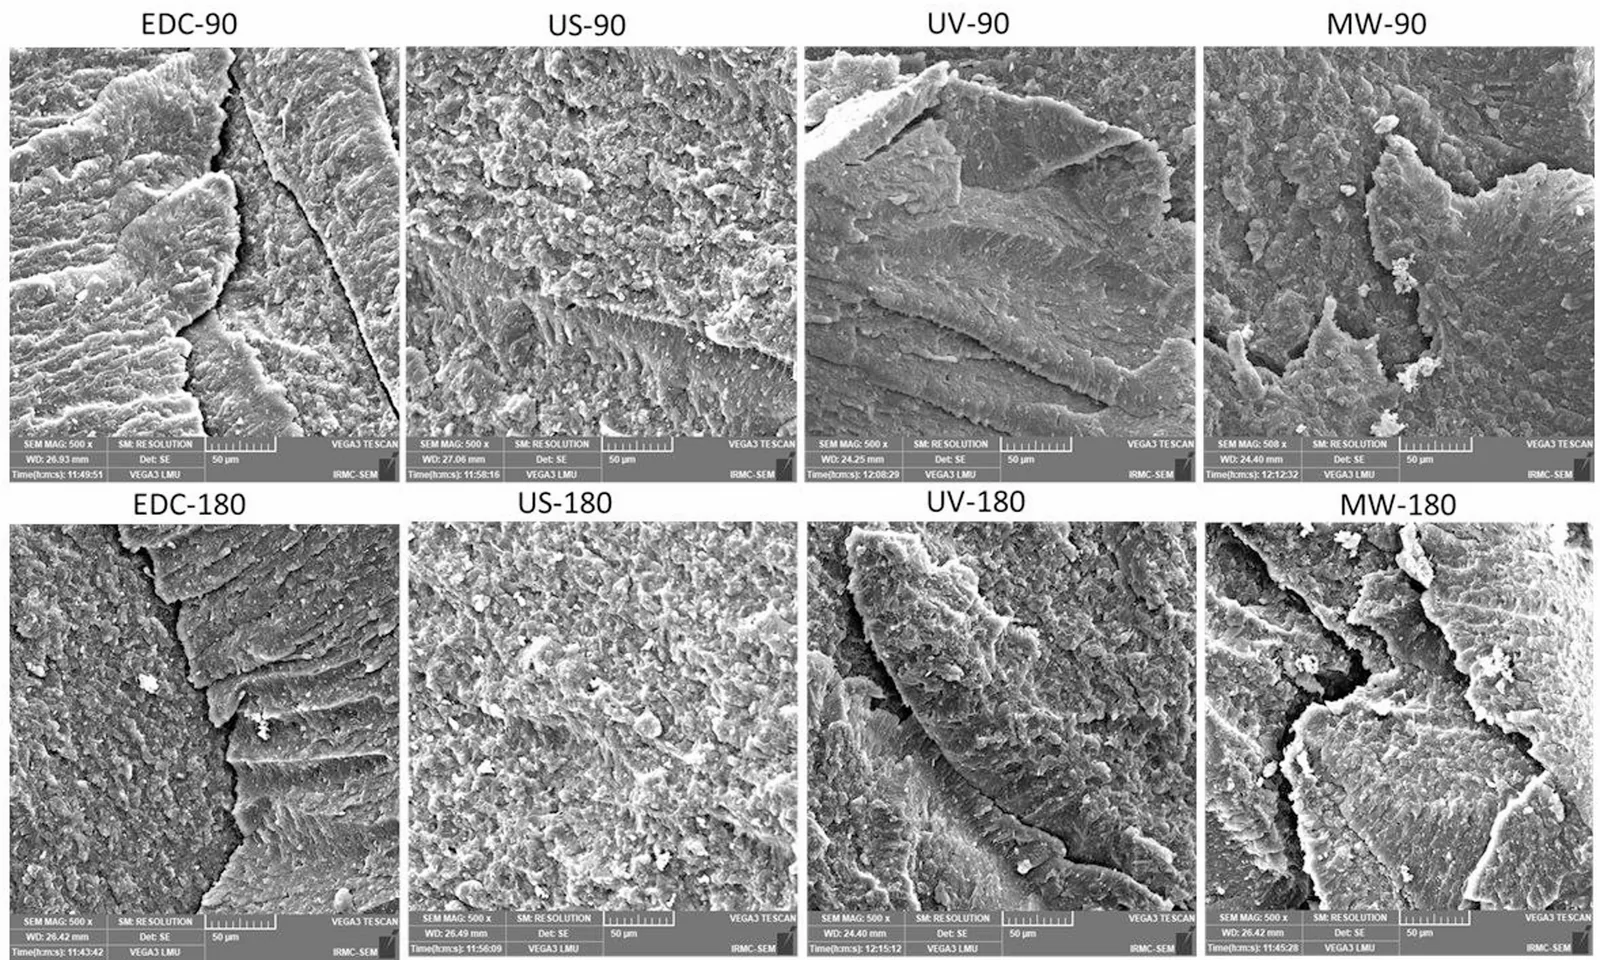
SEM images of disinfection groups for Denture 3D+ under x500 (ESC) Effervescent denture cleanser tablets (US), Ultrasonic, (UV) Ultraviolet, (MW) Microwave
